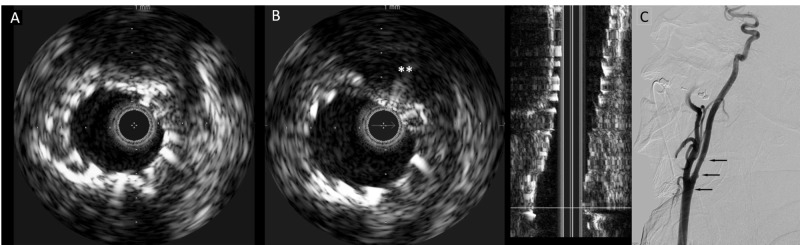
Intravascular ultrasound and digital subtraction carotid angiogram of Case 1 (A) Poststenting intravascular ultrasound image demonstrating good apposition of the stent to the vessel wall. (B) Thrombus is shown to be trapped (asterisks) by the deployed stent, without evidence of debris inside the stent. (C) Final lateral projection angiogram demonstrating significantly improved vessel caliber (arrows)
